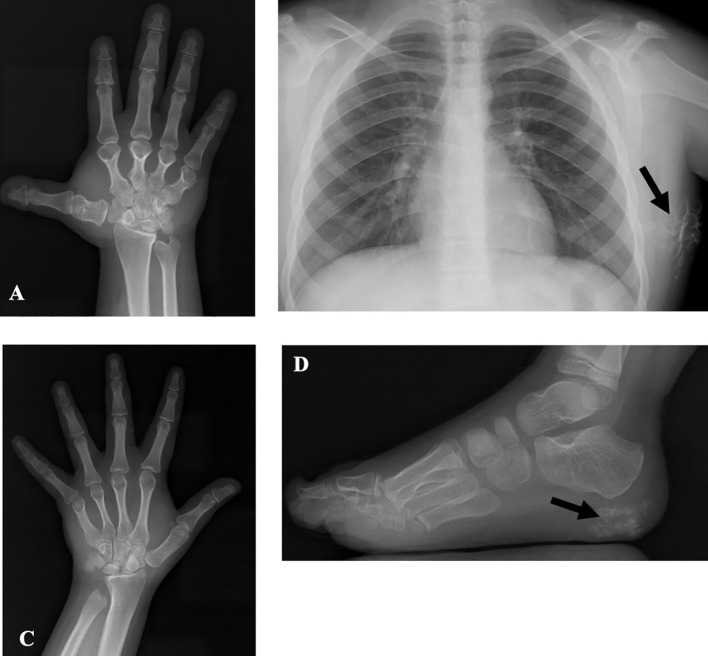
(A) Left hand radiograph of case II-1 demonstrating *brachydactyly* and *Madelung deformity*. (B) Posteroanterior chest radiography of case II-1, which demonstrates *osteoma cutis* of the left chest wall. (C) Left hand radiograph of Case III -2 showing severe *Madelung deformity.*
